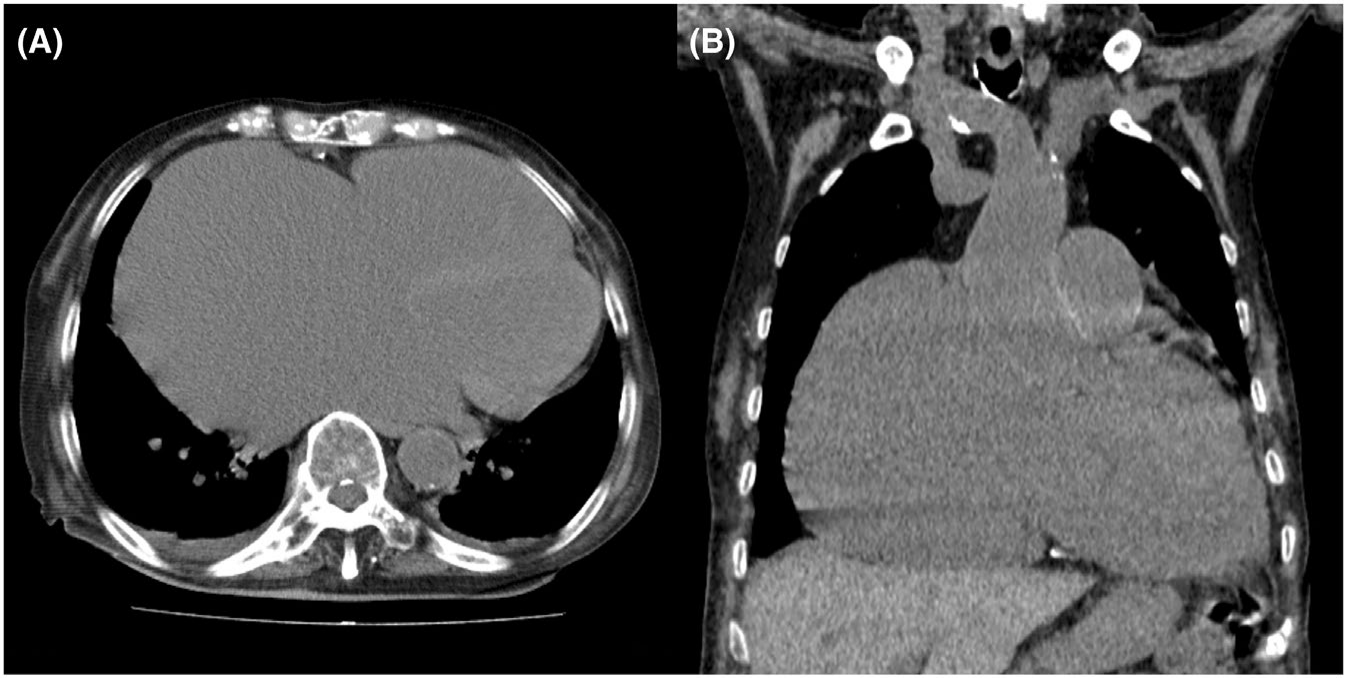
Computed tomography images indicating severe enlargement of the right atrium. (A) Axial view. (B) Coronal view.
